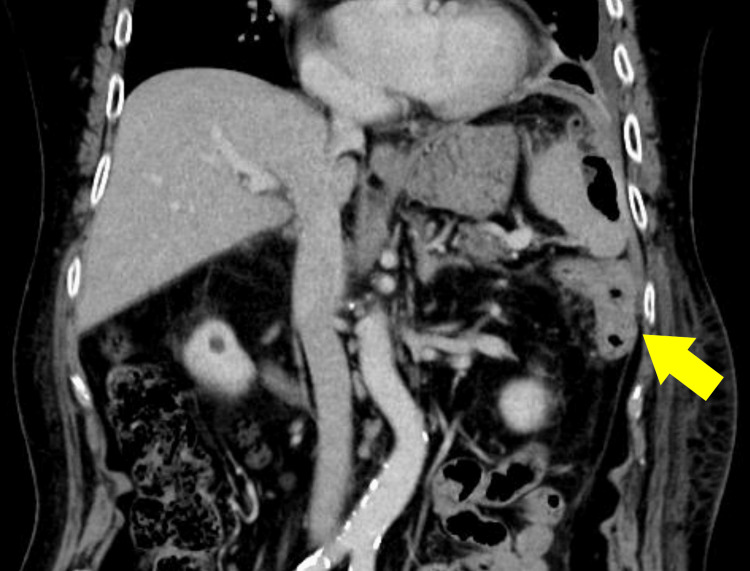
Contrast-enhanced CT at presentation showing wall thickening at the splenic flexure. Wall thickening with contrast enhancement was seen at the splenic flexure of the colon (yellow arrow).
